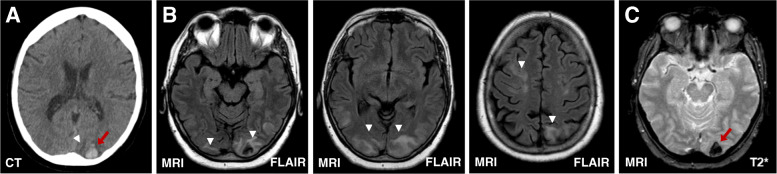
Vasogenic edema and occipital haemorrhage in PRES. a The initial CT scan detected a left located occipital haemorrhage (red arrow) as well as occipital accentuated subcortical hypodensities (white arrow head) indicative of an edema. b MRI scan confirmed the CT hypodensities as edema (white arrow heads) and the haemorrhage in the T2*-weighted gradient echo (c) (red arrow)
